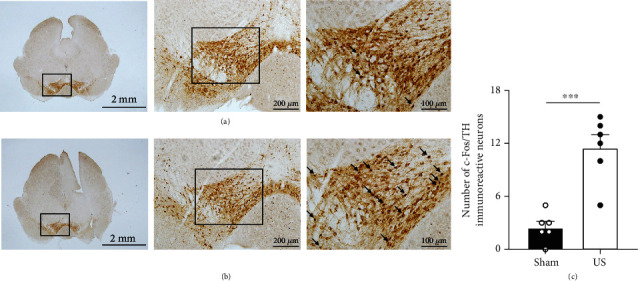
Ultrasound stimulation increases the abundance of c-Fos-positive neurons in the VTA. Representative c-Fos expression of the VTA in (a) the sham group and (b) the US group. Double labelling of TH (brown) and c-Fos (black) in the VTA. Arrows indicate c-Fos-positive cells. (c) Number of c-Fos-positive cells in the US group, showing a significant increment compared to that in the sham group. c-Fos-positive cells costained with dopamine neurons were counted in the entire VTA region of the brain slice (*n* = 6, mean ± SEM, ^∗∗∗^*p* < 0.001, independent-sample *t*-test).
